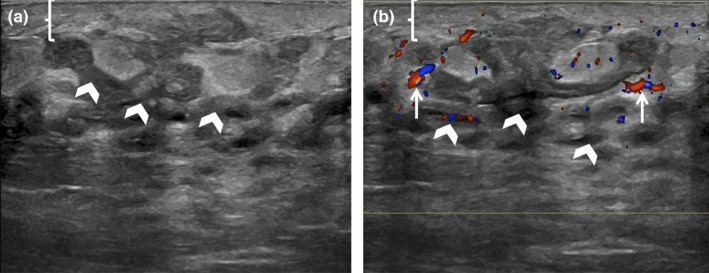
38‐year‐old lactating woman presented with concerns about redness of the left periareolar breast. Greyscale (a) and Color Doppler (b) Ultrasound was performed with a ML6‐15 MHz transducer with the frequency set to 11 MHz, and the images were captured from the skin to the chest wall. Targeted ultrasound evaluation at 6 o'clock 2 cm from the nipple demonstrated mild skin thickening measuring 4 mm (bracket), linear hypoechoic regions of interstitial fluid (chevrons) and associated hypervascularity (arrows). No discrete drainable fluid collection was identified. Constellation of findings was consistent with left breast mastitis with phlegmon. The patient was instructed to continue breastfeeding on a frequent basis, with warm compresses and massage in between, and to complete her 10‐day course of Keflex. Her symptoms were fully resolved, and she did not require a follow‐up ultrasound.
